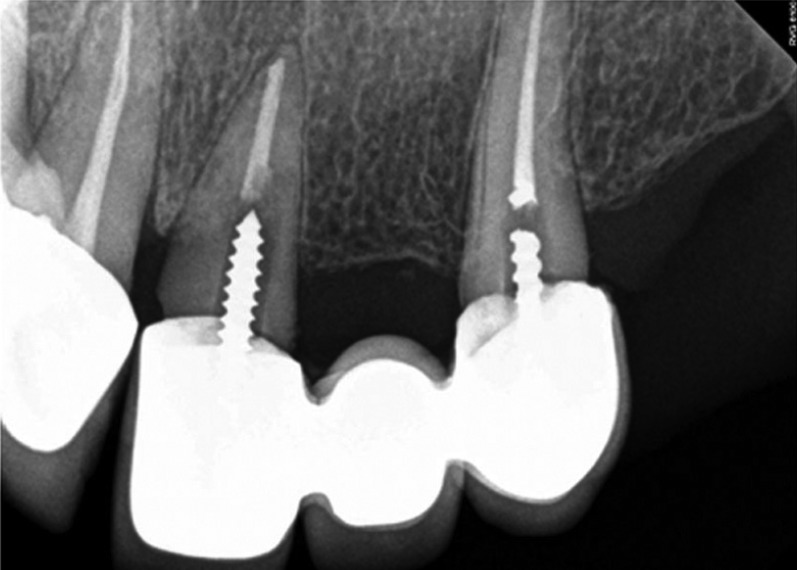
Radiographic picture of a failed FDP as a result of failed posts.
